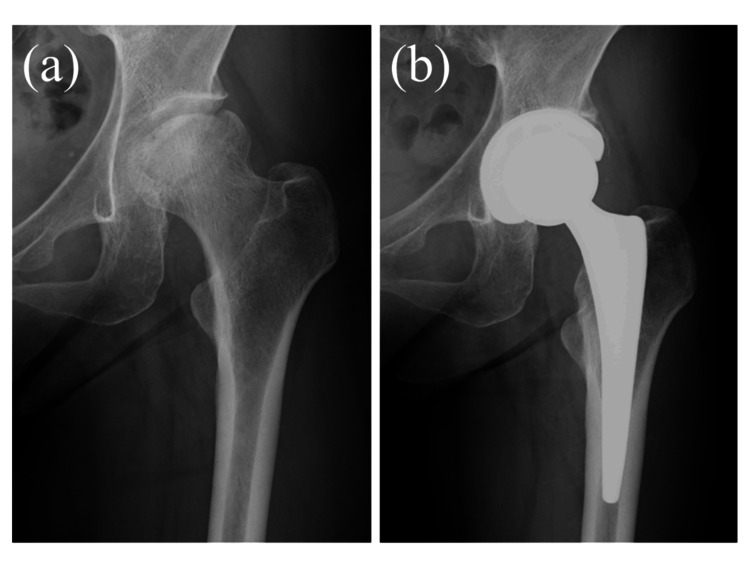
Preoperative anteroposterior radiograph (a) and postoperative anteroposterior radiograph at 12 months (b) of the superior approach for a patient with osteonecrosis of the femoral head
